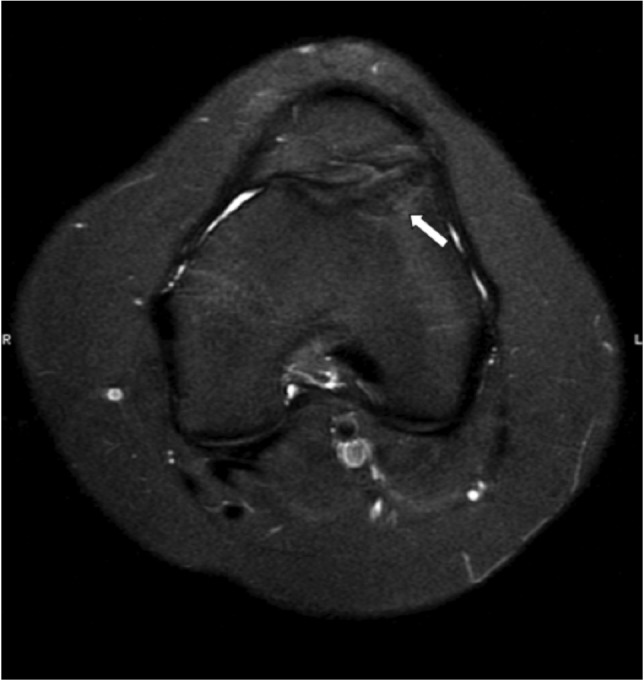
Postoperative magnetic resonance image demonstrates resolution of the bone marrow edema and continuity of the articular cartilage (white arrow).
